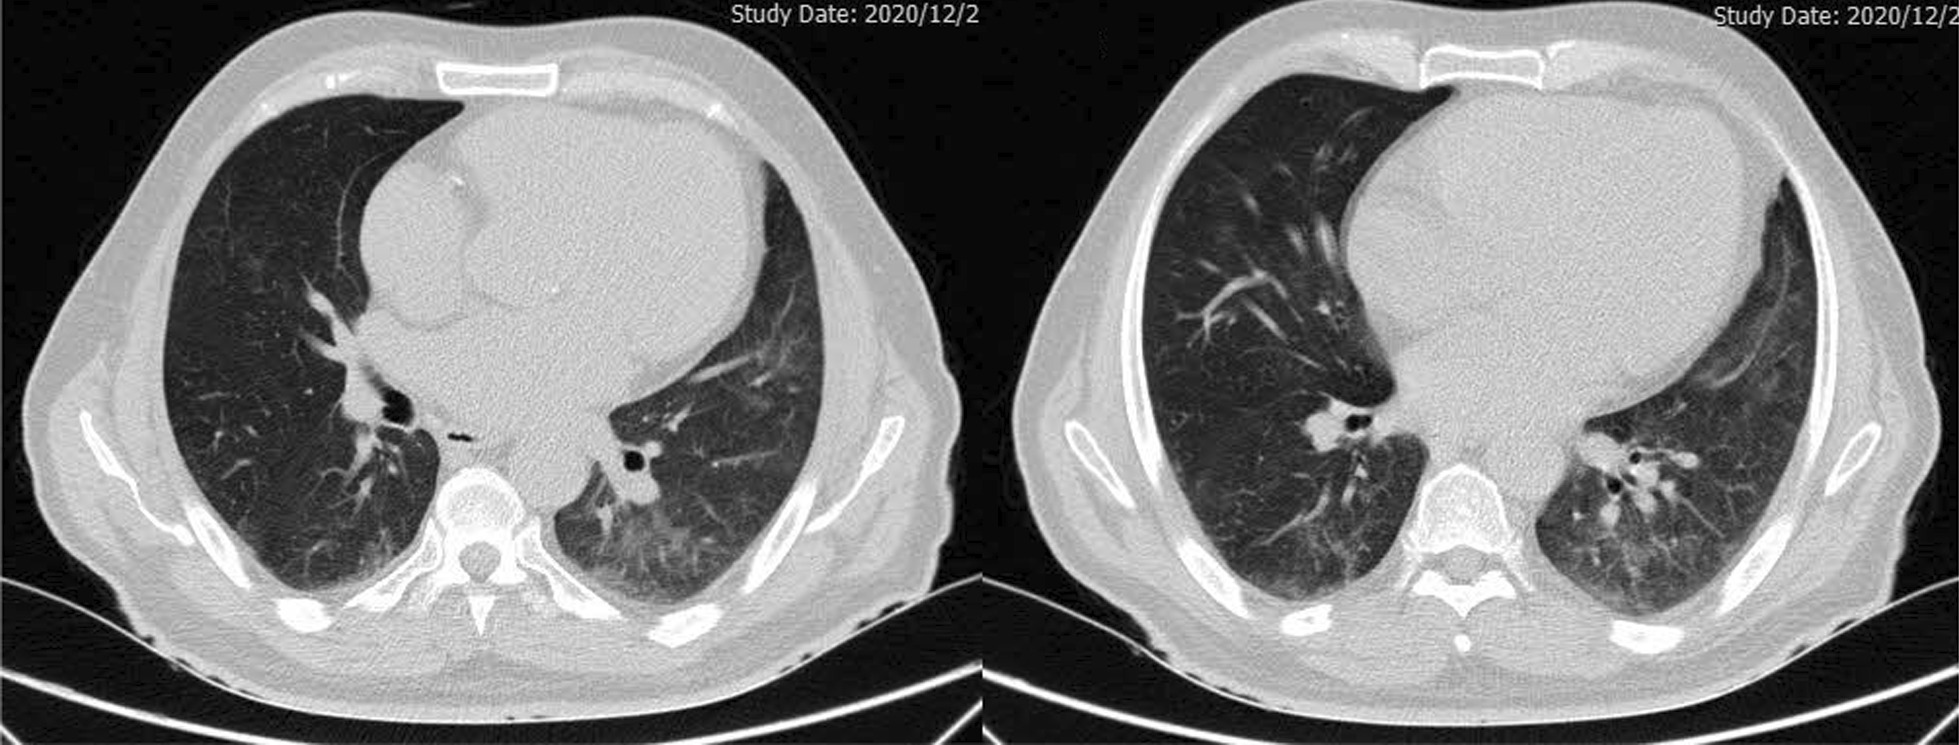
Chest high resolution computed tomography scan (without Contrast, Axial view) of a 67-year-old male patient with necrotizing autoimmune myositis following COVID-19 infection, demonstrating ground glass opacity in basal parts of the lung along with mild pericardial effusion
